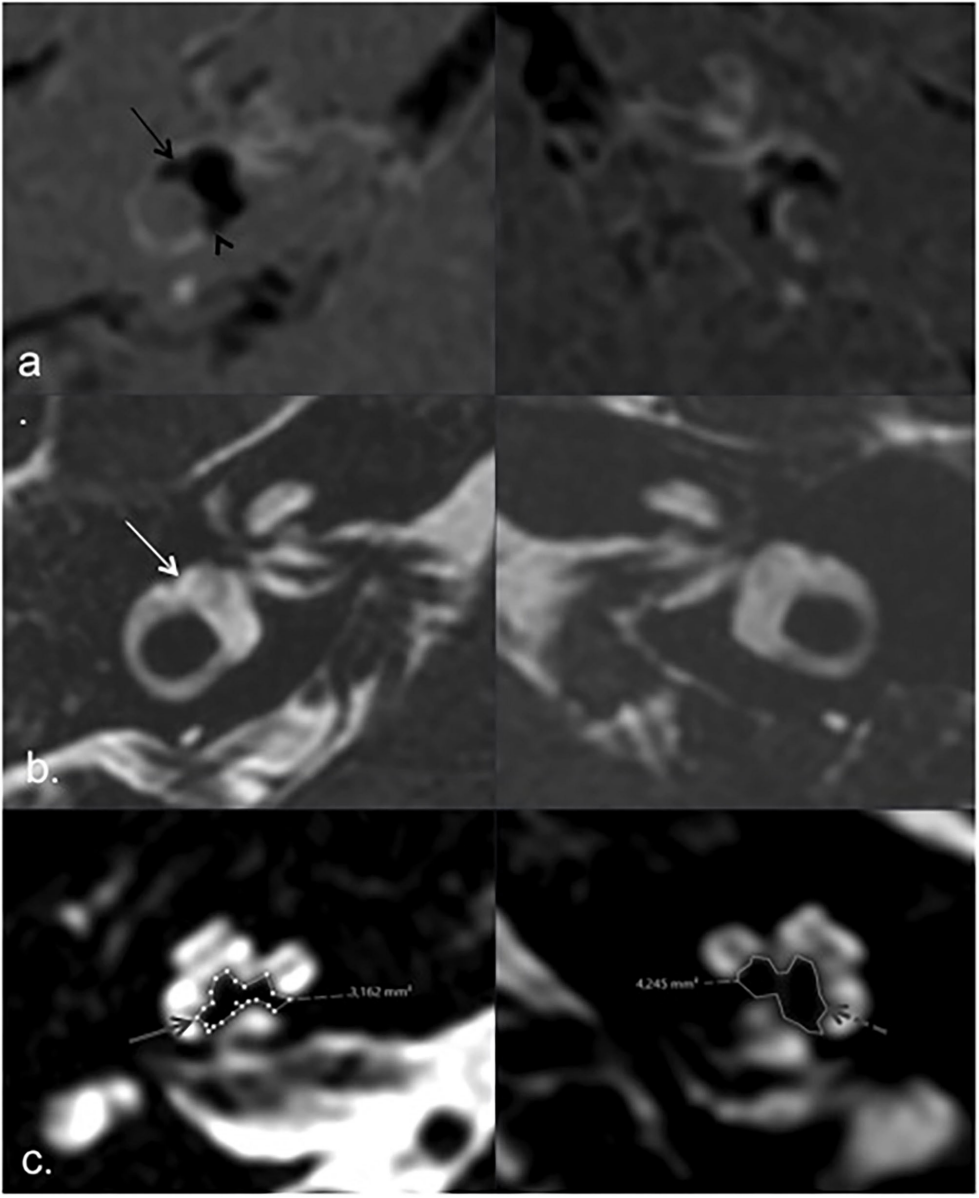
MRI of patient 2. HYDROPS (a) and T2 3D TSE (b) axial images according to lateral semicircular canal plane, showing endolymphatic (black arrows) and total vestibular labyrinth (white arrow) respectively. Extreme right vestibular endolymphatic hydrops with herniation of endolymph into de ampullary (black arrow) and non-ampullary (black arrowhead) endings of the lateral semicircular canal in right ear (index ear). Axial T2 image according to the greater axis of cochlear modiolus (c), showing marked asymmetric modiolar areas, smaller on the right side (solid gray arrow) compared to the left asymptomatic side (dashed gray arrow).
